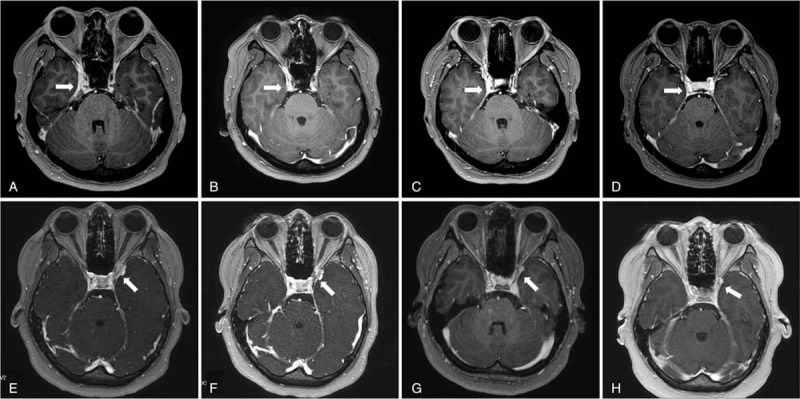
Serial gadolinium enhanced axial T1-weighted magnetic resonance images (MRI) of the patients (case 1: A–D, case 2: E–H). Enlargement and enhancement of right cavernous sinus and right superior orbital fissure was detected initially in 32-year-old woman (arrow, A). Two months later, lesion decreased markedly (B). Five months later, newly developed similar lesion was noted (C) and this lesion showed improvement 17 months later (D). MRI of 22-year-old woman showed small sized infiltrative mass at left superior orbital fissure (arrow, E). This lesion slightly decreased 2 months later (F). After second recurrence, more decreased lesion was seen (G). This lesion decreased more 8 months later, after adding methotrexate (H).
